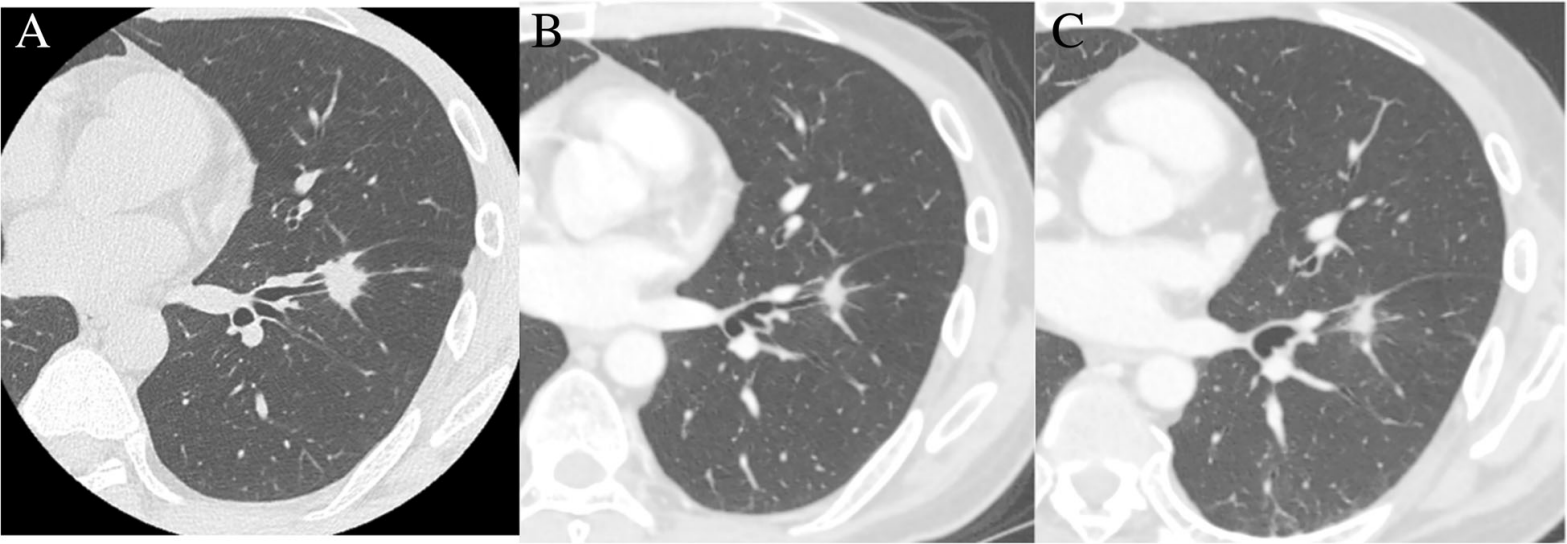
Chest computed tomography at first visit (a), 40 days after start of alectinib treatment (b), and 90 days after start of alectinib treatment (c)
